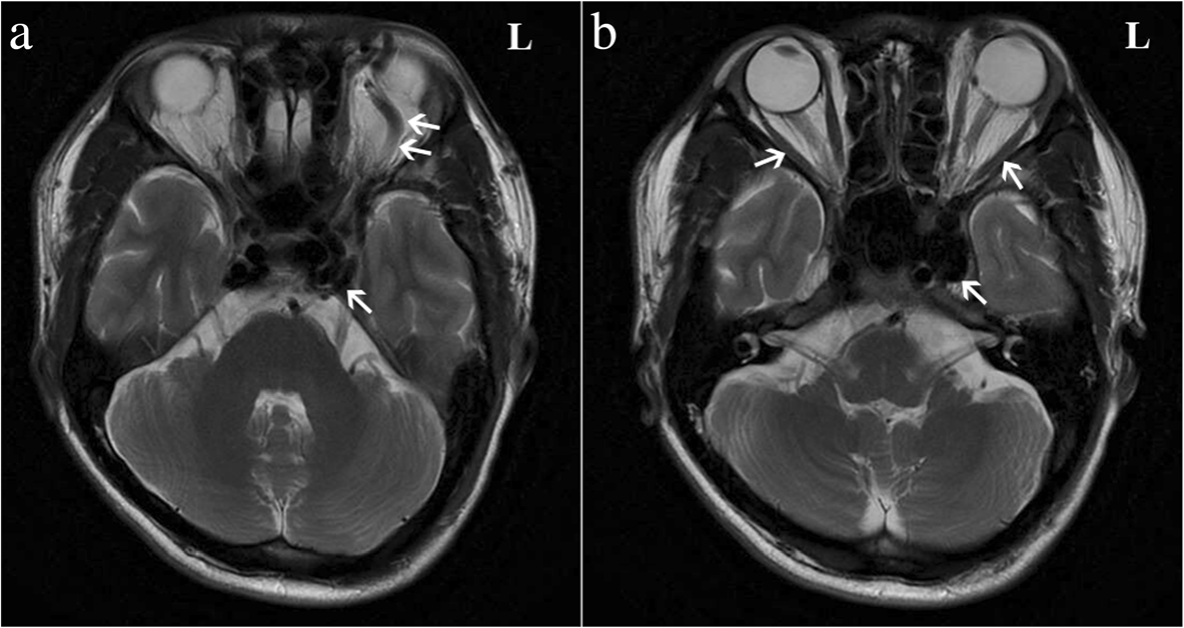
Magnetic resonance imaging (MRI) of the periorbital region. a. It revealed expansion of the left cavernous sinus and dilation of the left superior ophthalmic vein (white arrow). b. It revealed slight thickening of the left lateral rectus muscle and enlarged left cavernous sinus, yet the right was almost normal (white arrow).
